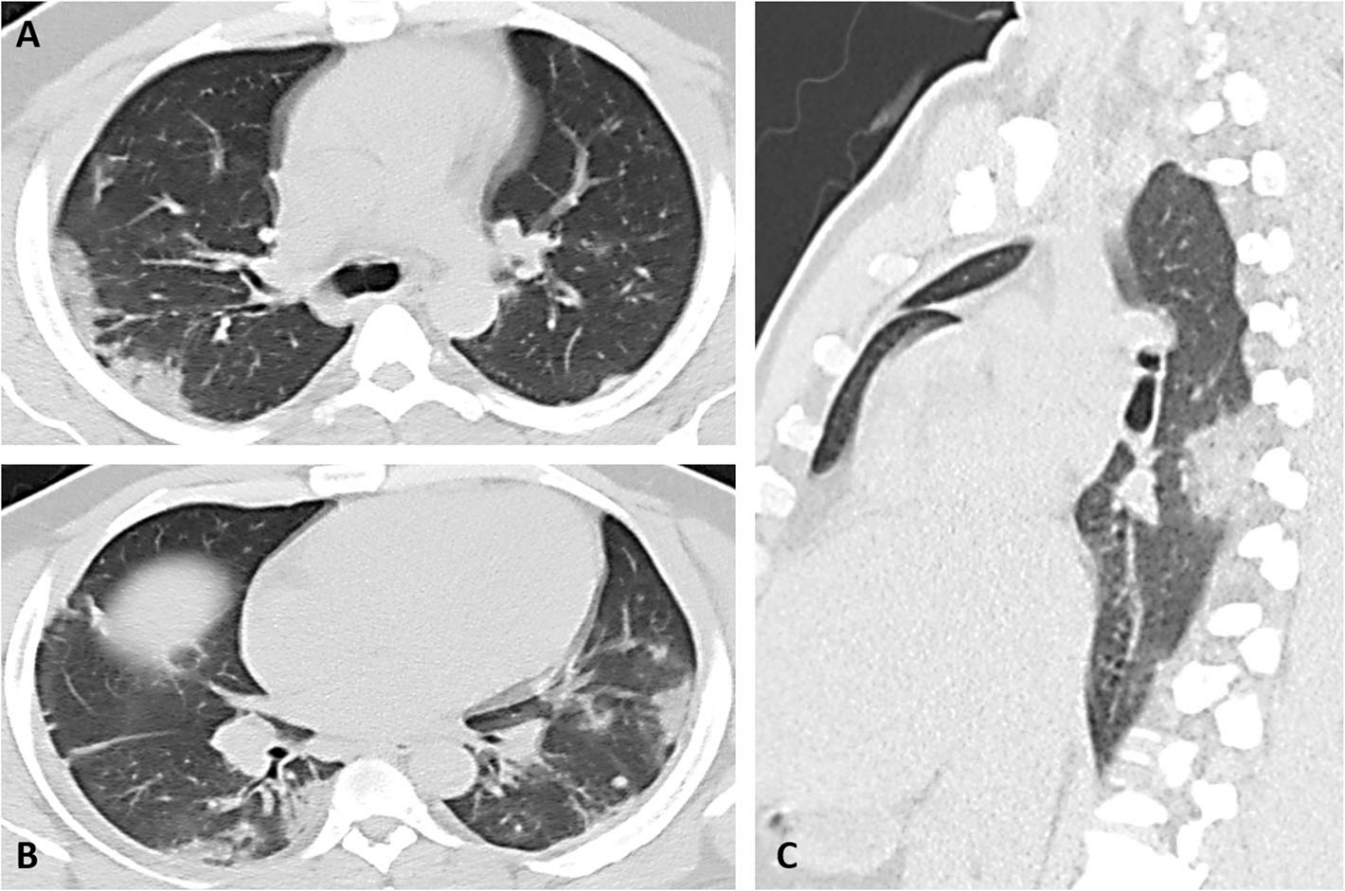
Non-contrast CT of a 52-year-old male with fever and shortness of breath, rt RT-PCR +VE (A-C): A and B axial, and C sagittal non-contrast CT scan lung window show bilateral multiple subpleural areas of consolidation associated with linear opacities and pleural thickening
